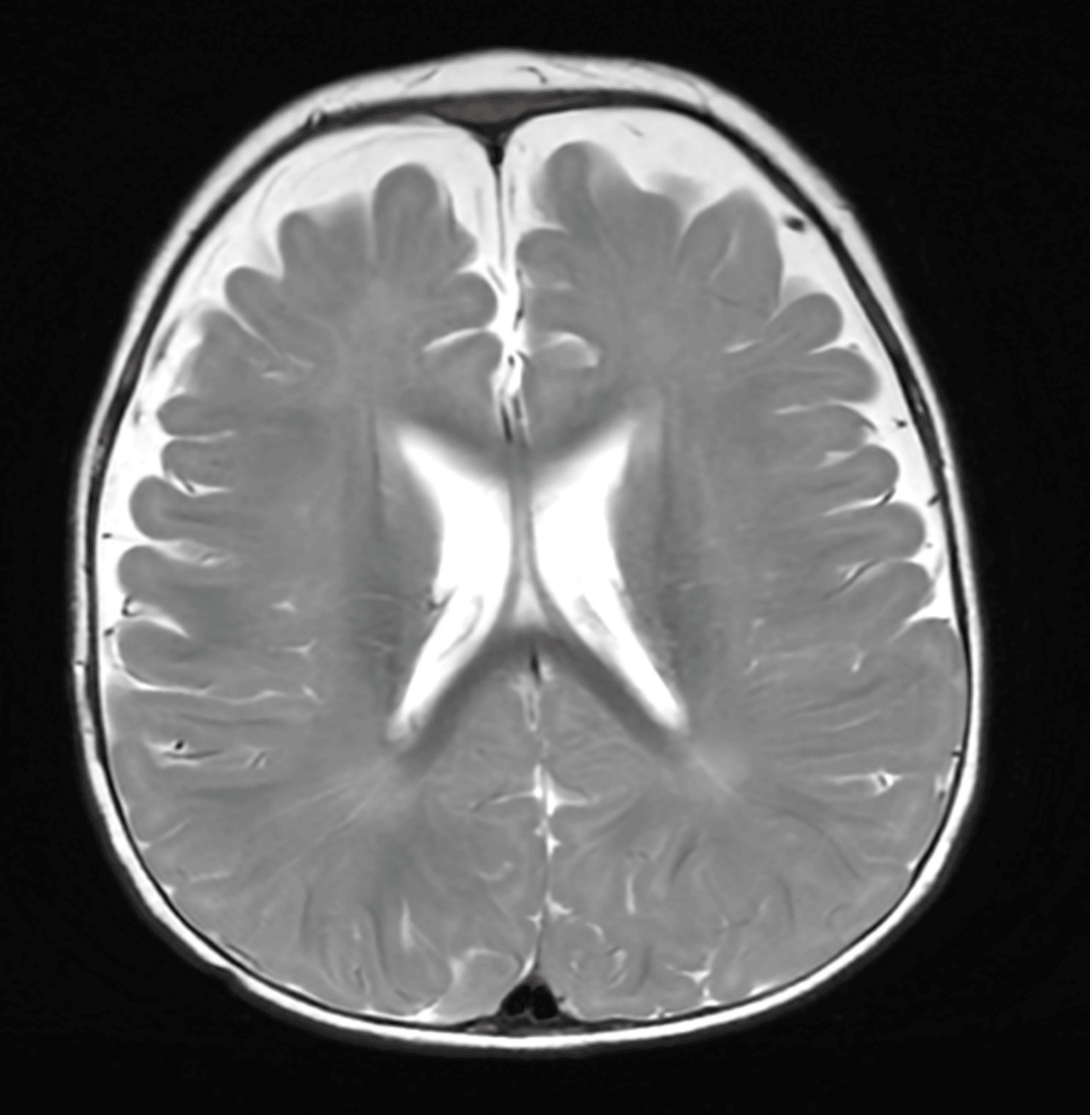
Brain MRI (Axial T2-weighted fast spin-echo) performed at eight months of age demonstrating diffuse, symmetric widening of the extra-axial CSF spaces over the frontal and parietal convexities, with preserved cortical morphology and normal ventricular size, consistent with BESS BESS: benign enlargement of subarachnoid spaces; CSF: cerebrospinal fluid
